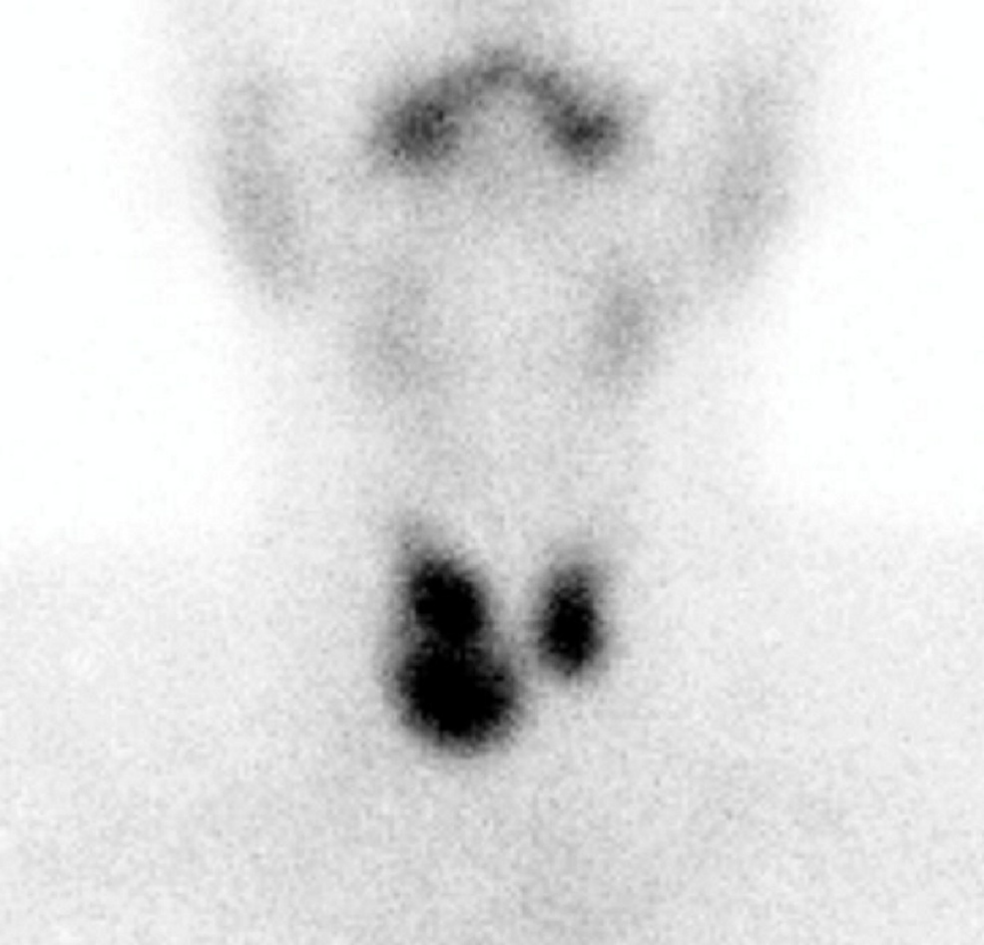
Thyroid scintigraphy Thyroid scintigraphy shows enlarged TL, with an increase in the Tc99m entrapment index; three hyper-uptake nodules are distinguished, two in the right TL and one in the left TL, without inhibiting the uptake of the rest of the gland tissue.
